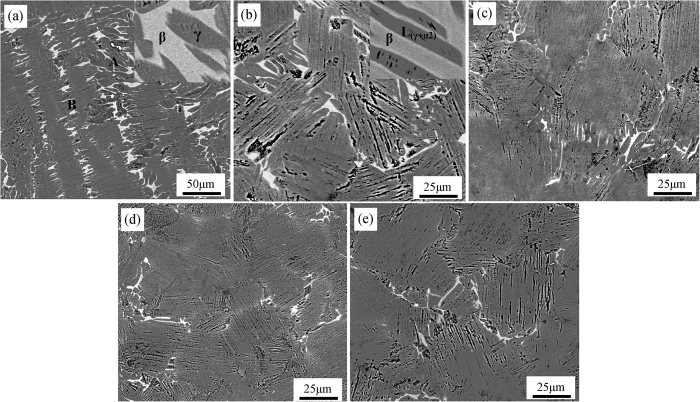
Effects of ultrasonic vibration time on the morphology and distribution of precipitated phases (B_2_). (a) 0 s; (b) 15 s; (c) 30 s, (d) 45 s, (e) 60 s.
